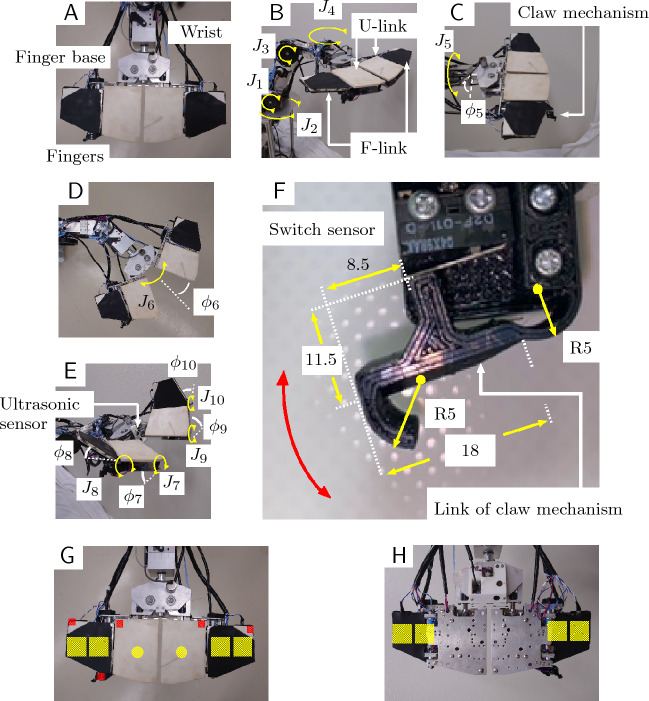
Details of the robotic hand mechanism. (A) Three parts of the hand: fingers, finger base, and wrist. (B) Axes of the manipulators. The shoulder axes are $$J_1$$ and $$J_2$$, and the elbow axes are $$J_3$$ and $$J_4$$. (C) Wrist axis rotating assembly, $$J_5$$, consisting of the finger base and fingers. One finger has a claw mechanism. (D) Axis set on the base controlling the angle of the fingers. The fingers are connected by multiple joints, which are driven by a single motor, $$\phi _6$$. (E) Axes that control the finger angle: $$J_7$$ to $$J_{10}$$. An ultrasonic sensor is installed on the base. (F) Approximate size of the claw mechanism. The mechanism was created with a 3D printer and is equipped with a touch sensor. (G) Positions of the switch sensor (small red squares) and the force (film) sensors on the front side of the finger (yellow circles and squares).(H) Positions of force (film) sensors on the back side of a finger (squares).
